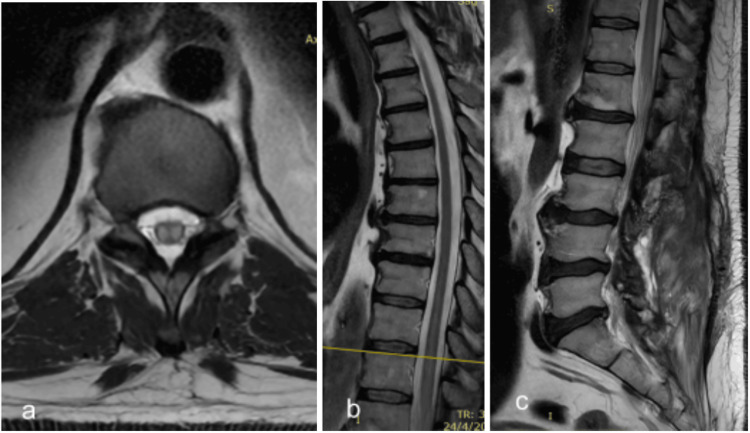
Three-month follow-up MRI of patient #1. a (axial T2WI) and b (sagittal T2WI): Oedema is still persistent in the sagittal T2WI and its respective level (marked with the yellow line in (b)) on the axial T2WI. c: T2WI shows no evident residual serpiginous flow voids.
